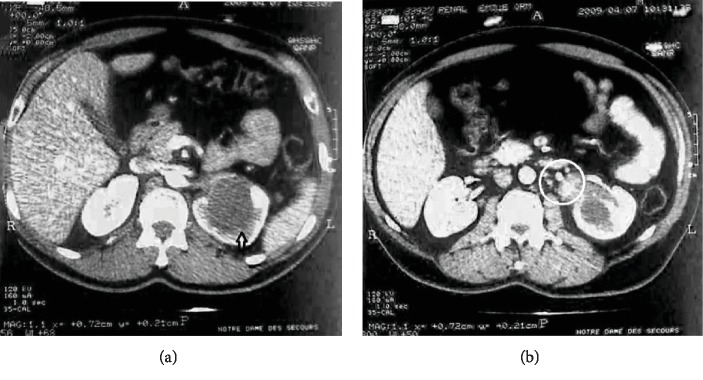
CT scan of the patient showing a 5 cm solid mass of the left renal pelvis (a, arrow) and multiple infracentimetric perihilar lymph nodes (b, circle).
